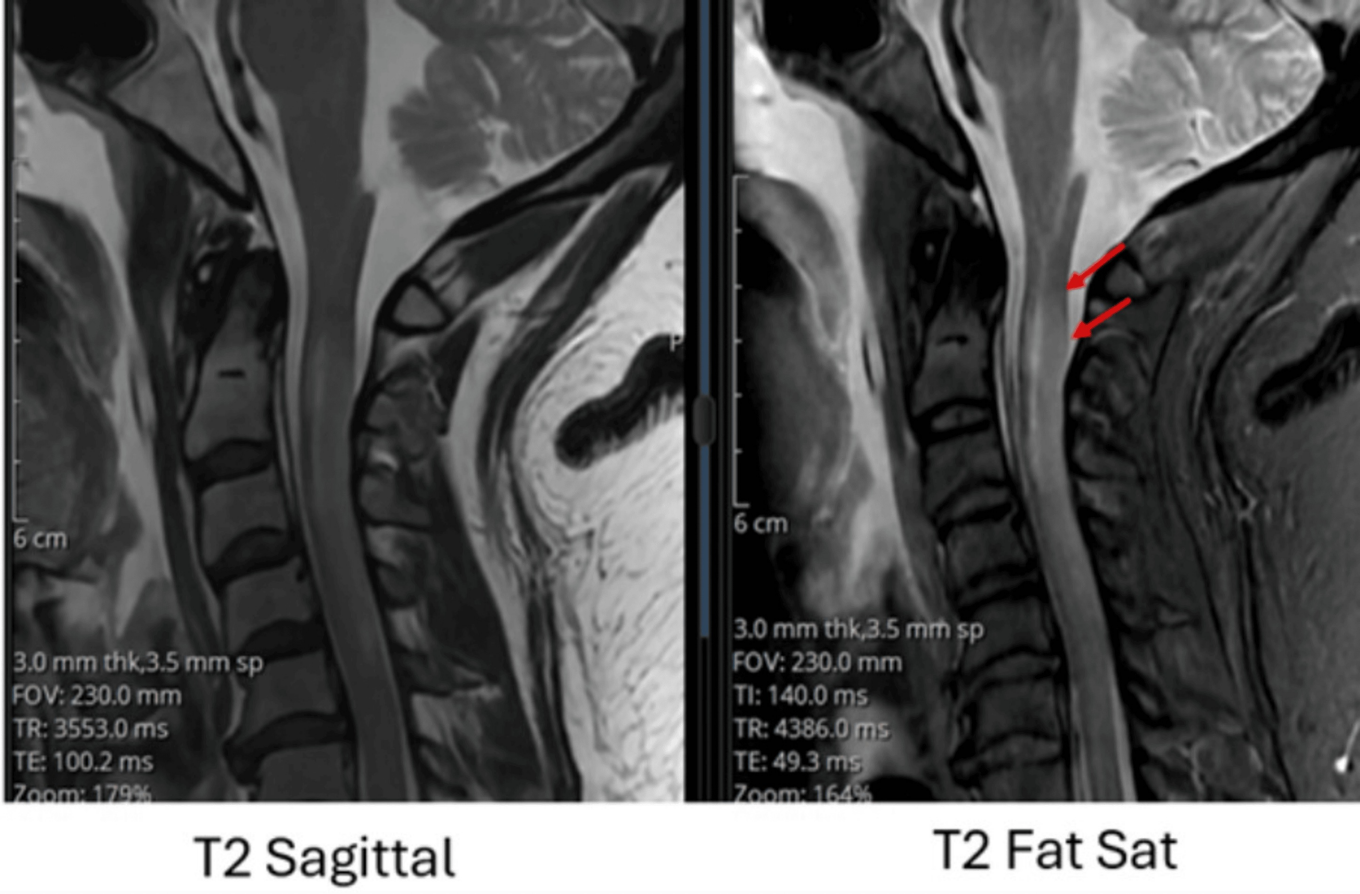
Progression of spinal cord hyperintensity on follow-up magnetic resonance imaging (MRI) of the cervical spine Follow-up sagittal T2-weighted and T2 fat-saturated MRI sequences of the cervical spine show increased conspicuity of the longitudinal hyperintense signal in the posterior spinal cord from C2 to C5 (red arrows), consistent with evolving spinal cord ischemia.
